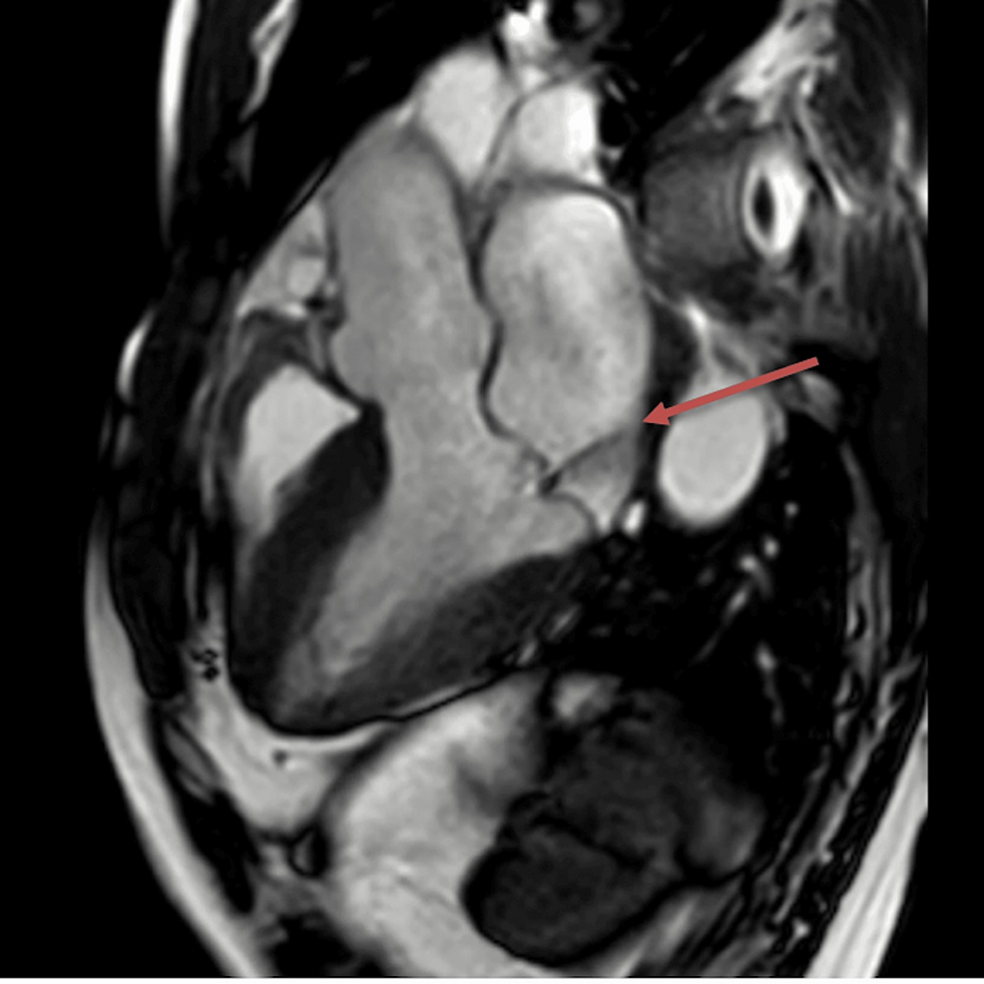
Cardiac MRI with an arrow indicating mild mitral valve regurgitation with posteriorly directed jet The regurgitant volume is 17 mL. The regurgitant fraction is 15%. MRI: magnetic resonance imaging
